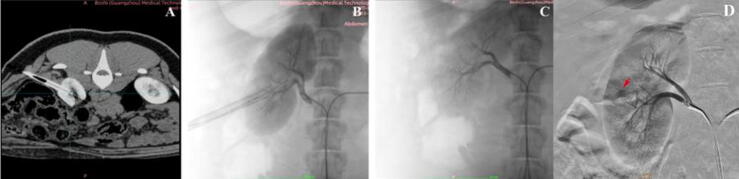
Postoperative CTA and DSA imaging: (A and B) dilated tract establishment confirmed by CTA and DSA, (C) assessment of vascular injury by DSA after tube removal in the D-CEUS–guided group, and (D) assessment of vascular injury in the conventional US-guided group. The red arrow indicates the bleeding site. CTA = computed tomographic angiography; D-CEUS = dual contrast-enhanced ultrasound; DSA = digital subtraction angiography; US = ultrasound.
